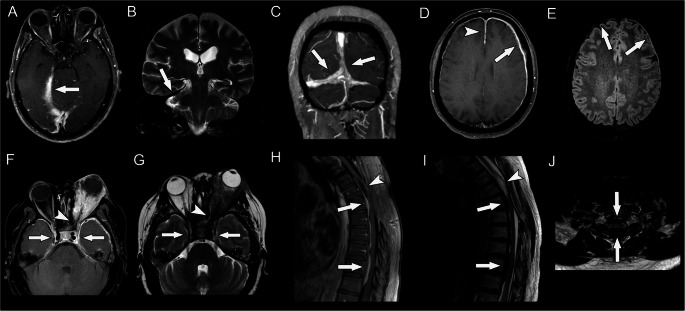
MR imaging findings in ANCA-associated pachymeningitis (patient 1, figures A-C; patient 2, figures D-E; patient 3, figures F-G; and patient 4, figures H-J). Axial postcontrast T1-weighted image (A) and coronal T2-weighted images (B) demonstrate dural thickening involving the right cerebellar tentorium, with contrast enhancement and T2-hypointensity (arrows). Coronal postcontrast T1-weighted image (C) shows extensive pachymeningeal involvement of tentorium and the posterior portion of the falx cerebri (arrows), resembling the so-called “Eiffel-by-Night” sign. Axial postcontrast T1-weighted (D) and FLAIR (E) images show pachymeningitis involving the frontoparietal convexity, more prominent to the right (arrows) and the anterior portion of the falx cerebri (arrowhead in D). Axial postcontrast T1-weighted image (F) and T2-weighted images (G) depict a large amount of a T2-hypointense tissue, with avid contrast enhancement, infiltrating the left orbit and causing proptosis Notice also the extension to the orbital apex (arrowheads) and both cavernous sinuses (arrows), together with dural thickening in the middle cranial fossa bilaterally, especially near the temporal poles. Sagittal postcontrast T1-weighted (H) and T2-weighted images (I) demonstrate dural thickening inside the thoracic vertebral canal with T2-hypointensity and contrast enhancement (arrows); there were also postoperative changes in the posterior portion of the upper thoracic spine together with myelopathy (arrowhead). Axial T2-weighted image of the same patient, in the cervicothoracic transition (J), shows spinal cord compression between anterior and posterior T2-hypointense pachymeningitis (arrows)
